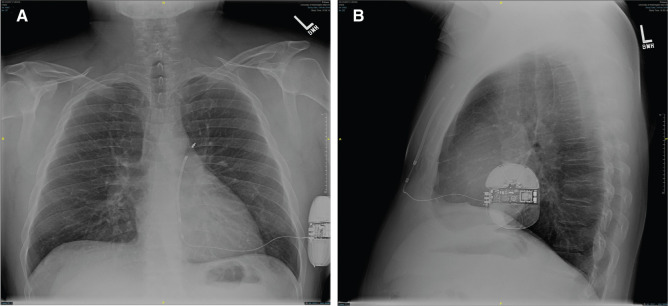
Postoperative posteroanterior (A) and lateral (B) chest X-rays demonstrating the position of the coil along the left parasternal border and the midaxillary position of the pulse generator.
